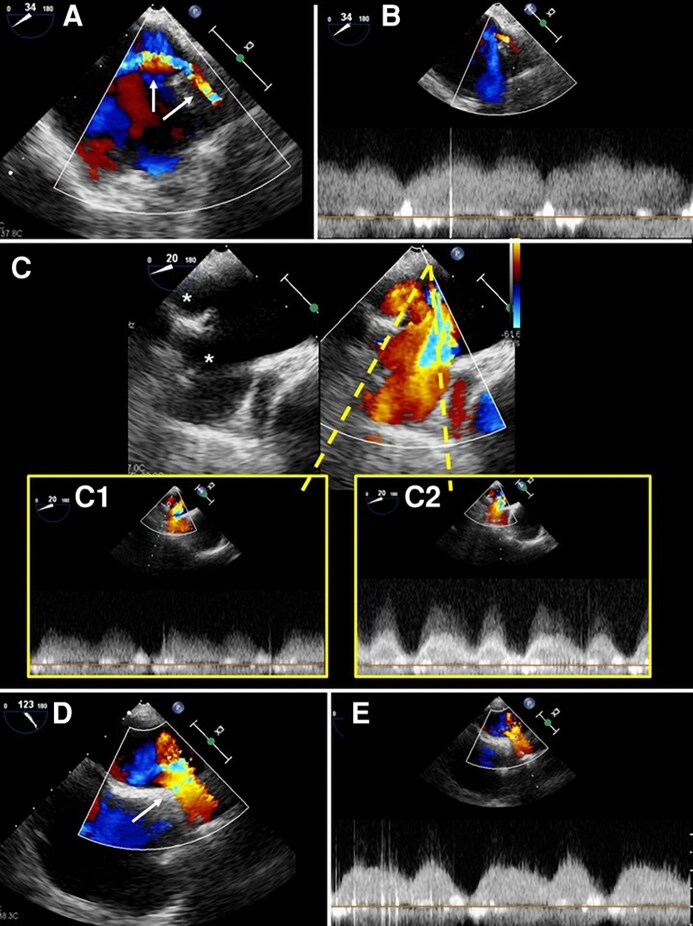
Flow acceleration and gradients across PV stenosis are assessed by positioning the pulsed-wave (PW) Doppler sample volume within the centre of the vein or at the level of maximal aliasing. Normally, pulmonary vein PW Doppler shows three waves: systolic (S), diastolic (D), and atrial reversal (Ar). Stenosis is considered significant when the maximum PV flow velocity exceeds 1.1 m/s. (*A*): 2D colour Doppler of the LUPV showing multicolour aliasing (white arrows) due to stenosis. (*B*): PW Doppler interrogation of the LUPV demonstrates high-velocity flow consistent with stenosis. (*C*): PW Doppler interrogation of the right PVs (asterisks), with higher velocities/gradient in panel C.2 compared with panel C.1. (*D*): 2D colour Doppler of the RUPV (from the ME modified bicaval view) showing aliasing (white arrow) due to stenosis. (*E*): PW Doppler recording with blunted systolic velocity and prolonged elevated diastolic velocity, with severe stenosis. LUPV: Left upper pulmonary vein; ME: Mid-oesophageal; PV: Pulmonary vein; RUPV: Right upper pulmonary vein.
